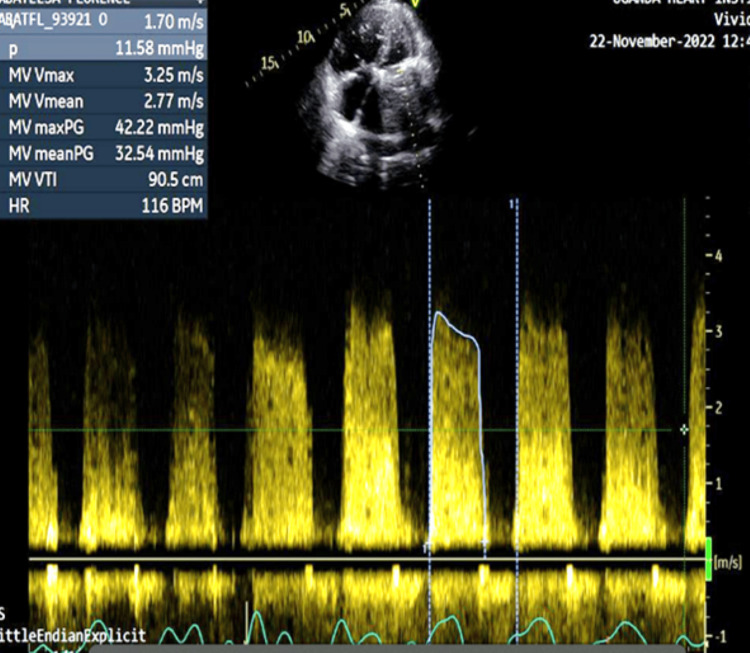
Spectral Doppler across the mitral prosthesis shows markedly elevated gradients
